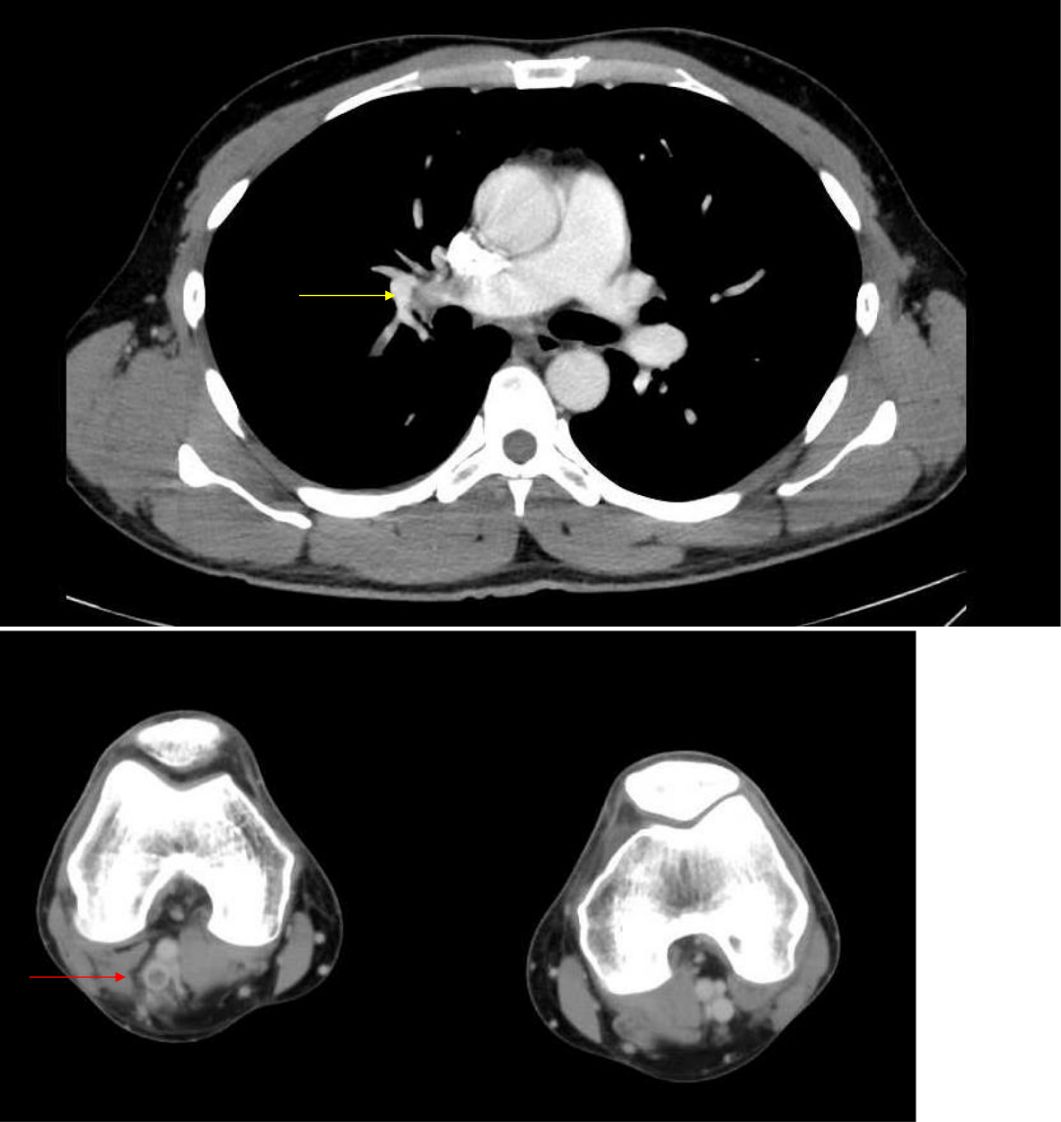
Contrast-enhanced computed tomography at the first visit. a A contrast deficit in the bilateral pulmonary artery (*yellow arrow* indicates the right pulmonary artery). b A contrast deficit in the left lower extremity (*red arrow*)
